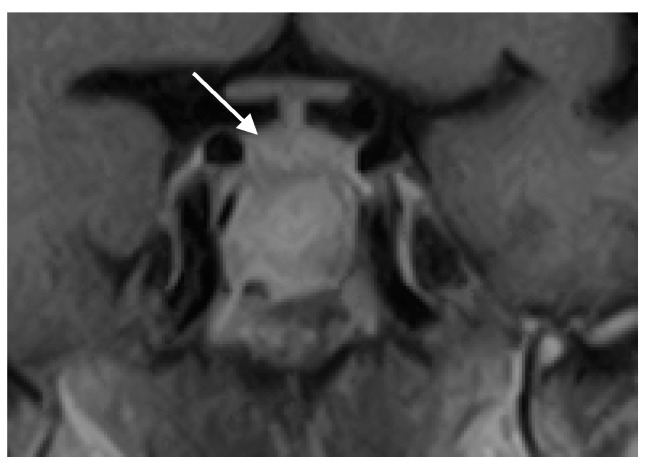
Coronal MRI scan of the sellar region after the operation showing a complete resolution of the pathological process and a normal pituitary gland (marked with white arrow).
